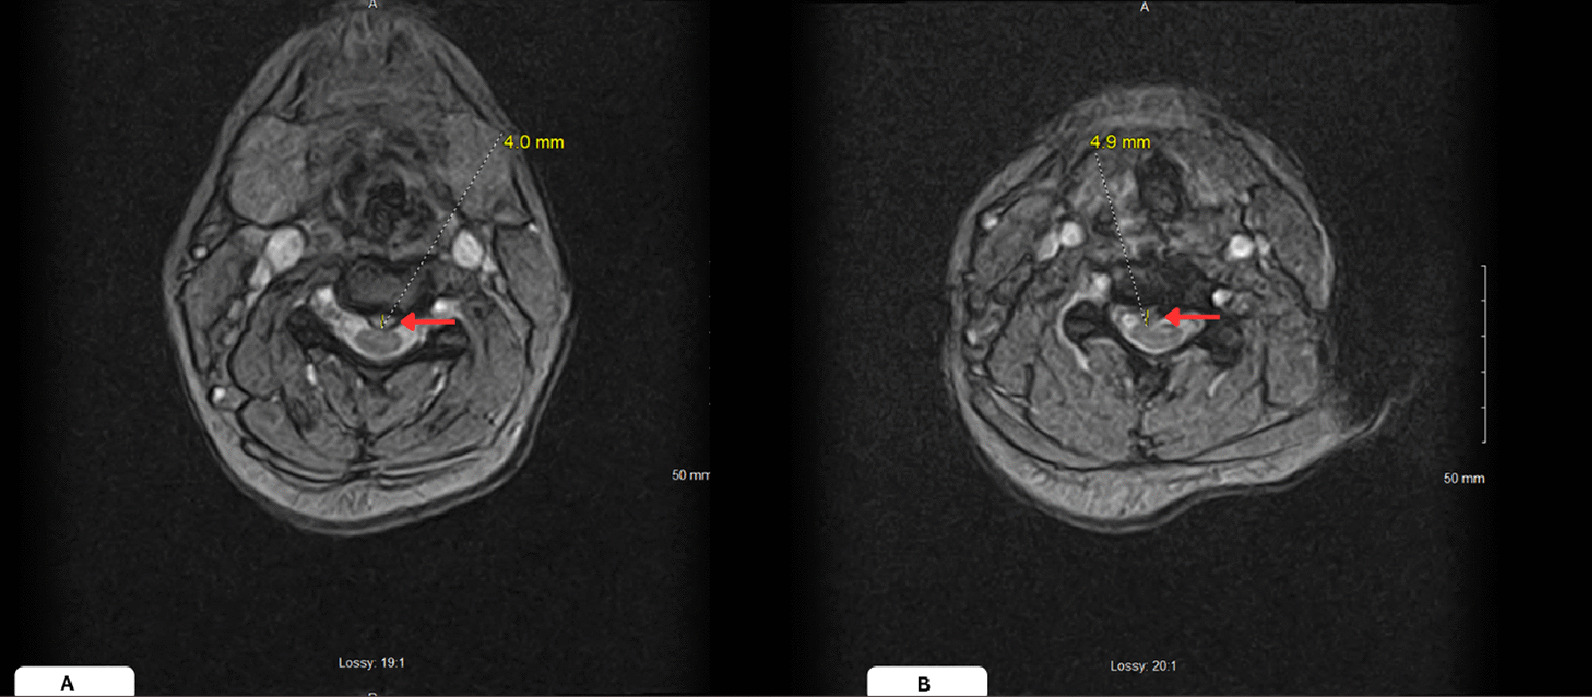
A Axial section of T2 weighted magnetic resonance imaging gradient echo displaying anterior cervical epidural hematoma at the level of C3 (left, red arrow). B Axial section of T2 weighted magnetic resonance imaging gradient echo displaying anterior cervical epidural hematoma at the level of C6 (right, red arrow)
